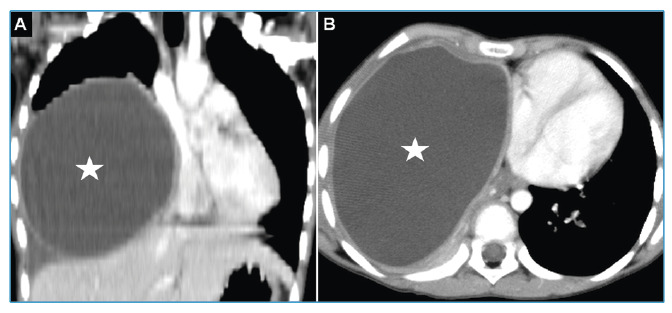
Contrast-enhanced thoracic CT coronal (A) and axial sections (B) shows a giant hydatic cyst of approximately 9 cm x 12 cm x 13 cm in the lower lobe of the right lung (arrows) and a shift to the left in the mediastinum.
